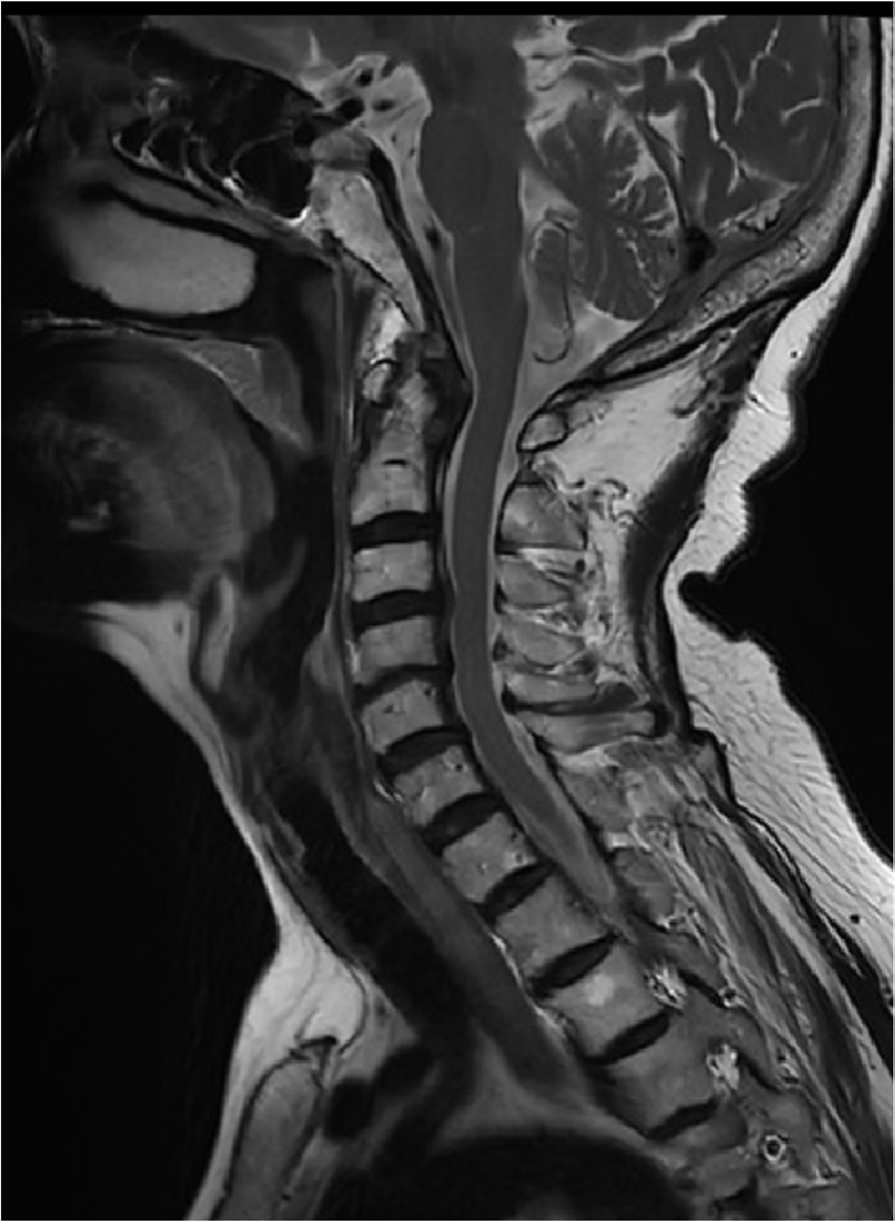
Sagittal MRI of cervical spine
